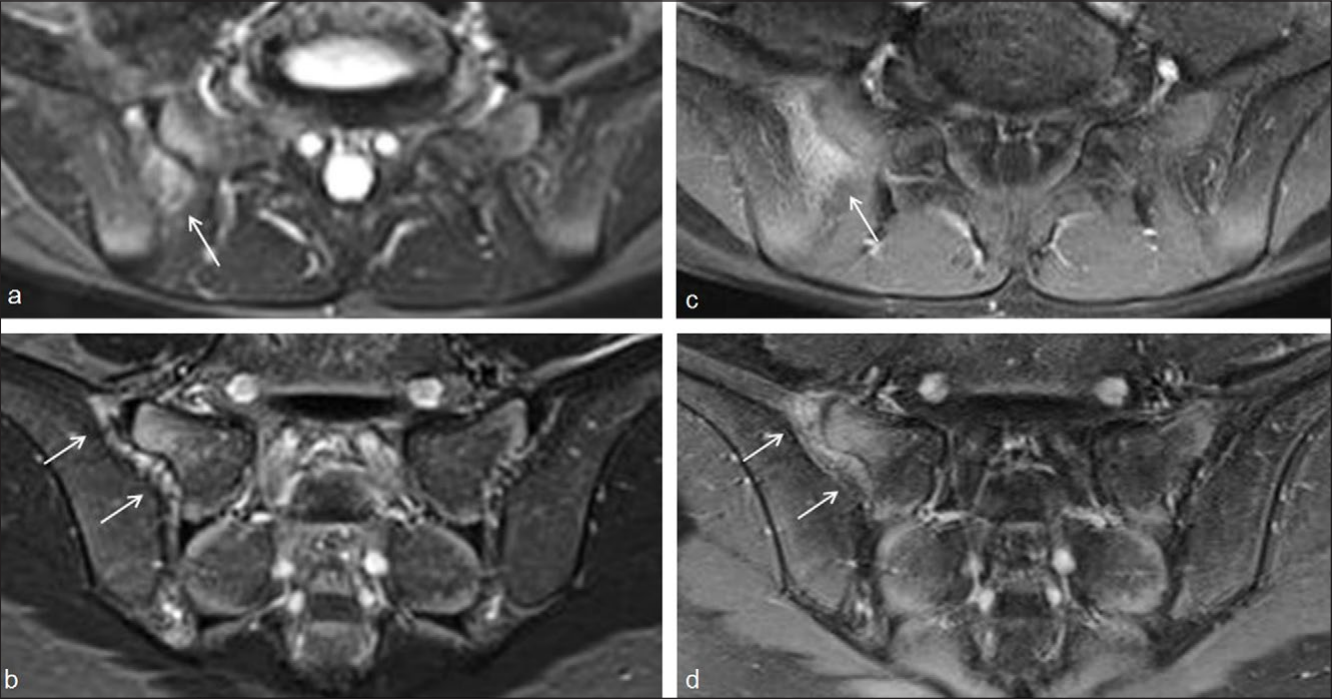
Active sacroiliitis with retroarticular enthesitis and capsulitis in a 14-year-old boy with JSpA: no added value of Gd. Axial and semicoronal STIR (a–b) and contrast-enhanced fat-saturated T1-weighted (c–d) images demonstrate enthesitis of the retroarticular interosseous ligaments and capsulitis on the right side (arrows).
